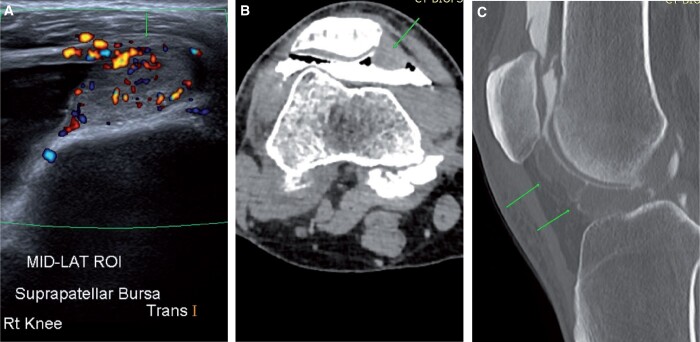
PVNS: (A) ultrasound image showing solid lesion (arrow) in the suprapatellar recess with internal vascularity. (B) Corresponding CT arthrogram image showing soft tissue thickening in the suprapatellar recess (arrow). (C) Extensive changes in the Hoffa’s fat pad (arrows) in the same knee.
